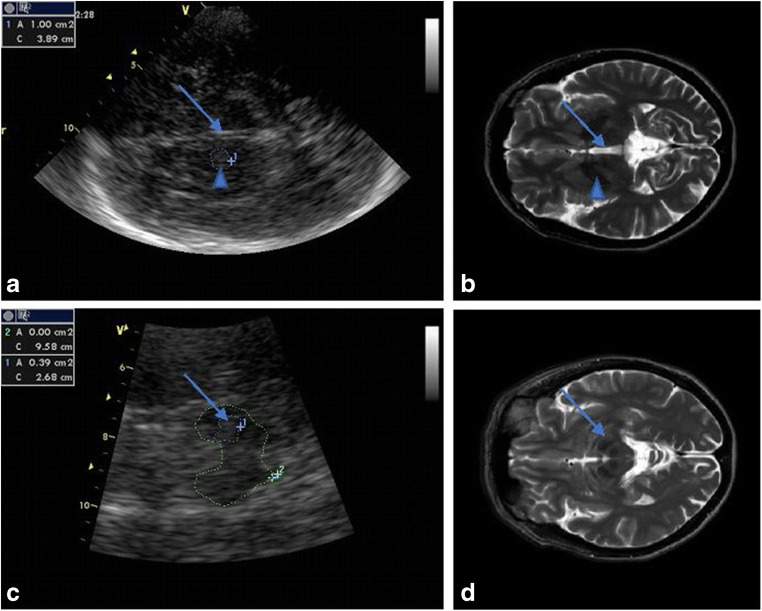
Midbrain structures in transcranial sonography (TCS) with corresponding magnetic resonance imaging (MRI) T2-weighted images. a TCS with the hyperechogenic part of the LN measured (arrowhead). Arrow showing the 3rd ventricle, b Corresponding MR results. c TCS with substantia nigra measured (arrow). d. Corresponding MR results
